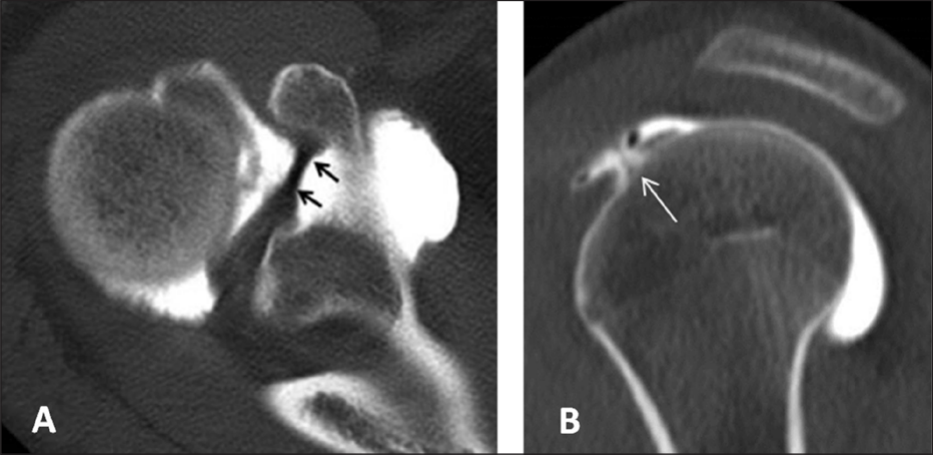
Superior glenohumeral ligament. It can be seen as a medium-size structure running almost straight from the superior labrum into the direction of the coracoid process on axial CTA (black arrows, (A). On sagittal CTA, the ligament appears as a T-shaped structure (thin white arrow, (B) Interposed between the long head of the biceps tendon posteriorly and the subscapularis tendon anteriorly.
